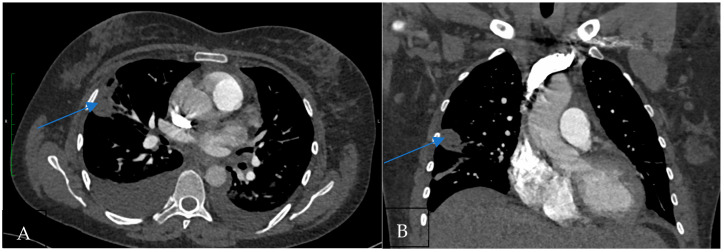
(A) CT pulmonary angiogram shows the wedge-shaped subpleural pulmonary infarction in the axial plane (Hampton’s hump; arrow). (B) CT pulmonary angiogram shows the wedge-shaped subpleural pulmonary infarction in the coronal plane.
